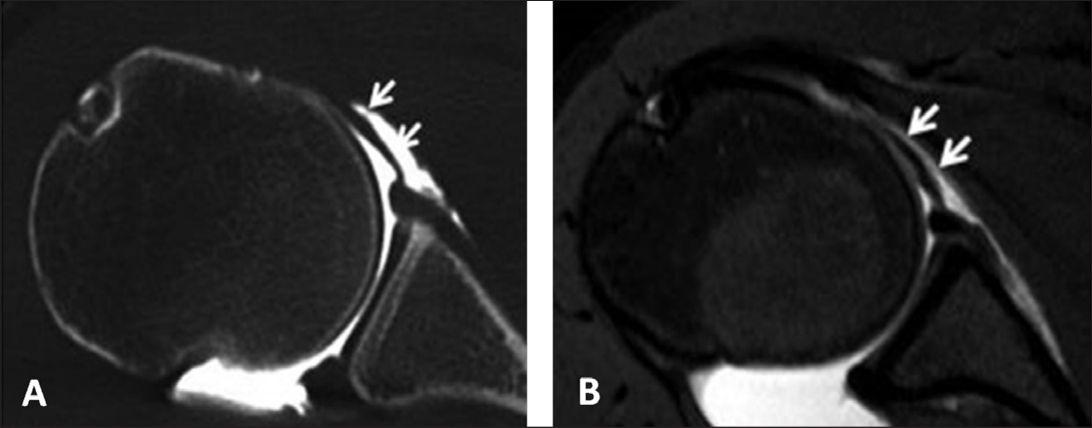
Middle glenohumeral ligament. The midsection of the ligament often adopts a more horizontal course. This can result in a thinner, wavier appearance on axial fat-saturated proton density MRA image (arrows, B) with a longer section of the ligament (arrows) on CTA (A).
